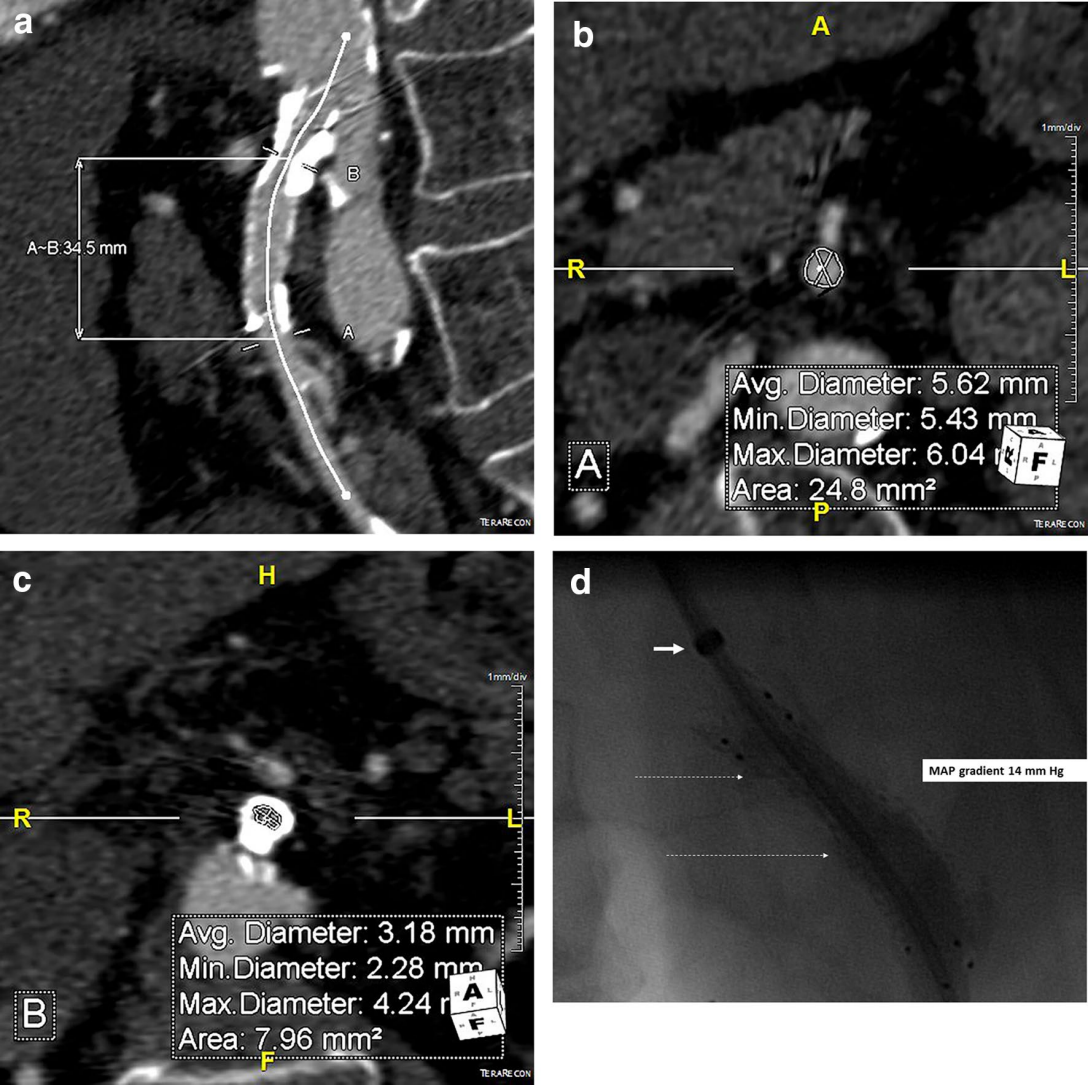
69-year-asymptomatic female patient with stented SMA. Follow-up colour doppler ultrasound showed a peak systolic velocity of 6.5 m/s. TeraRecon 3D CTA image indicates the centerline of flow (Fig. 1a). Measurements of arterial diameters were always performed in the plane perpendicular to its course. The respective average diameters were measured at the largest diameter distal to the occlusive lesion and the endoprosthesis (Fig. 1b) and narrowest intraluminal part of the stented arterial segment (Fig. 1c). SMA stent stenosis was calculated to be 43%. Angiography (Fig. 1d) was performed under general anaesthesia. The SMA was accessed percutaneously from the right brachial artery. The tip of the introducer (thick short arrow) ends at the origin of the SMA from the aorta. The hard atherosclerotic stenotic lesion at the backwall of the SMA (between dashed arrows) has caused a recoil of the endoprosthesis after previous stentgrafting (best visualized in this un-subtracted image), resulting in a MAP gradient of 14 mm Hg. After subsequent re-balloon dilatation, the MAP gradient was reduced to 5 mm Hg. Since then, the patient has remained symptom-and re-intervention-free during the seven-year follow-up
